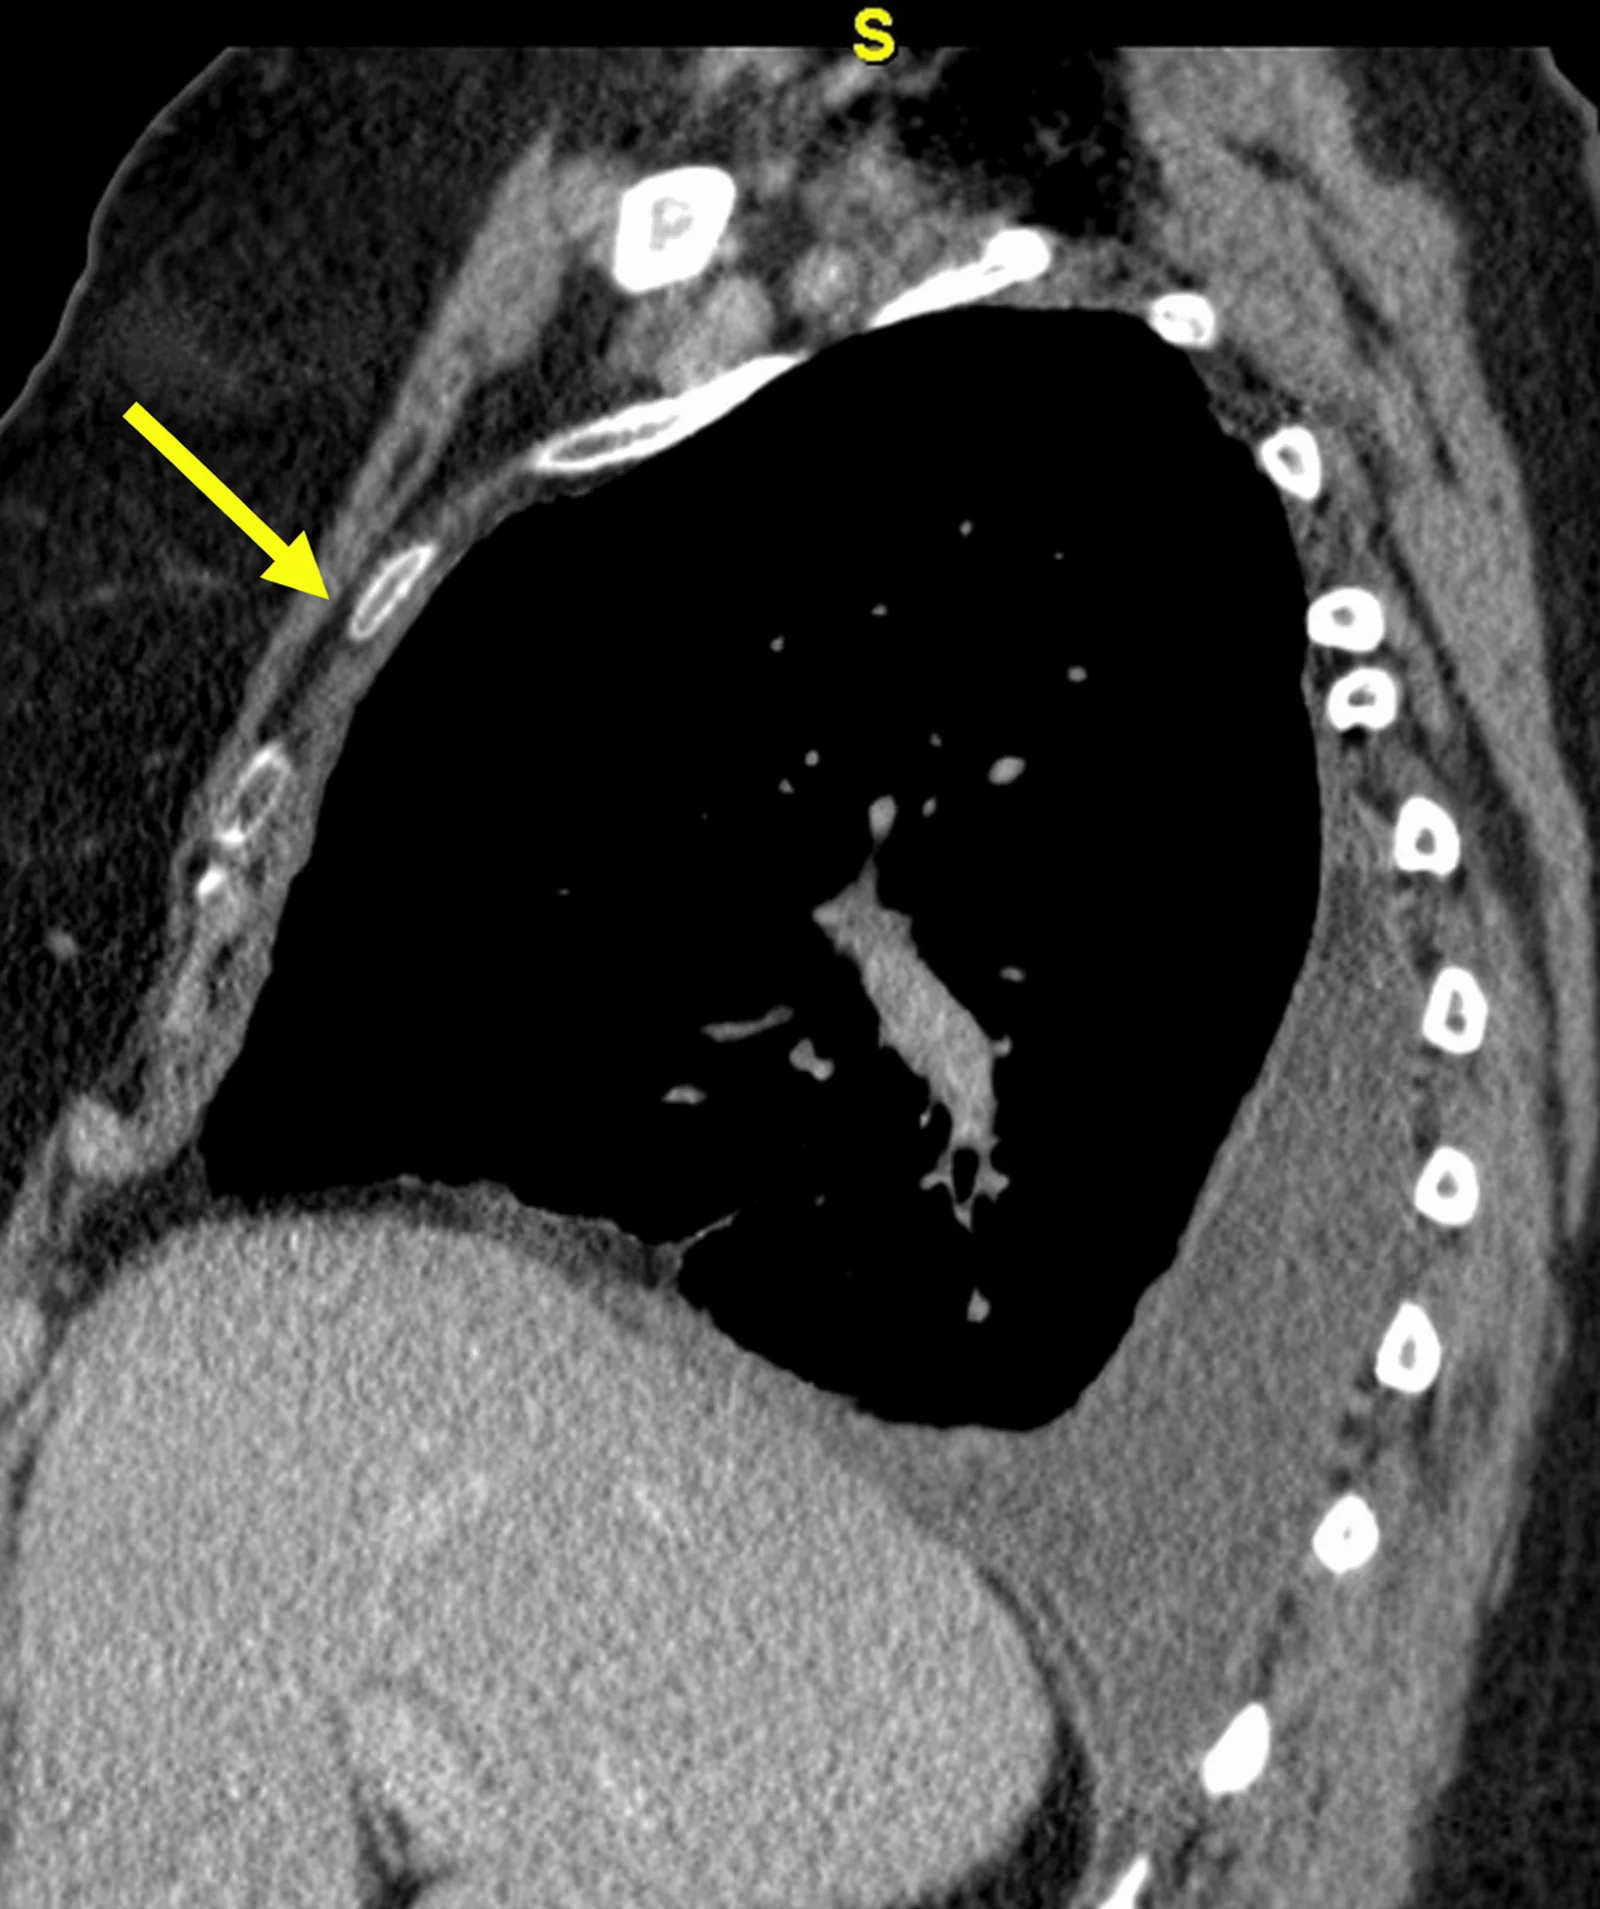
Sagittal CT demonstrating right pleural thickening
